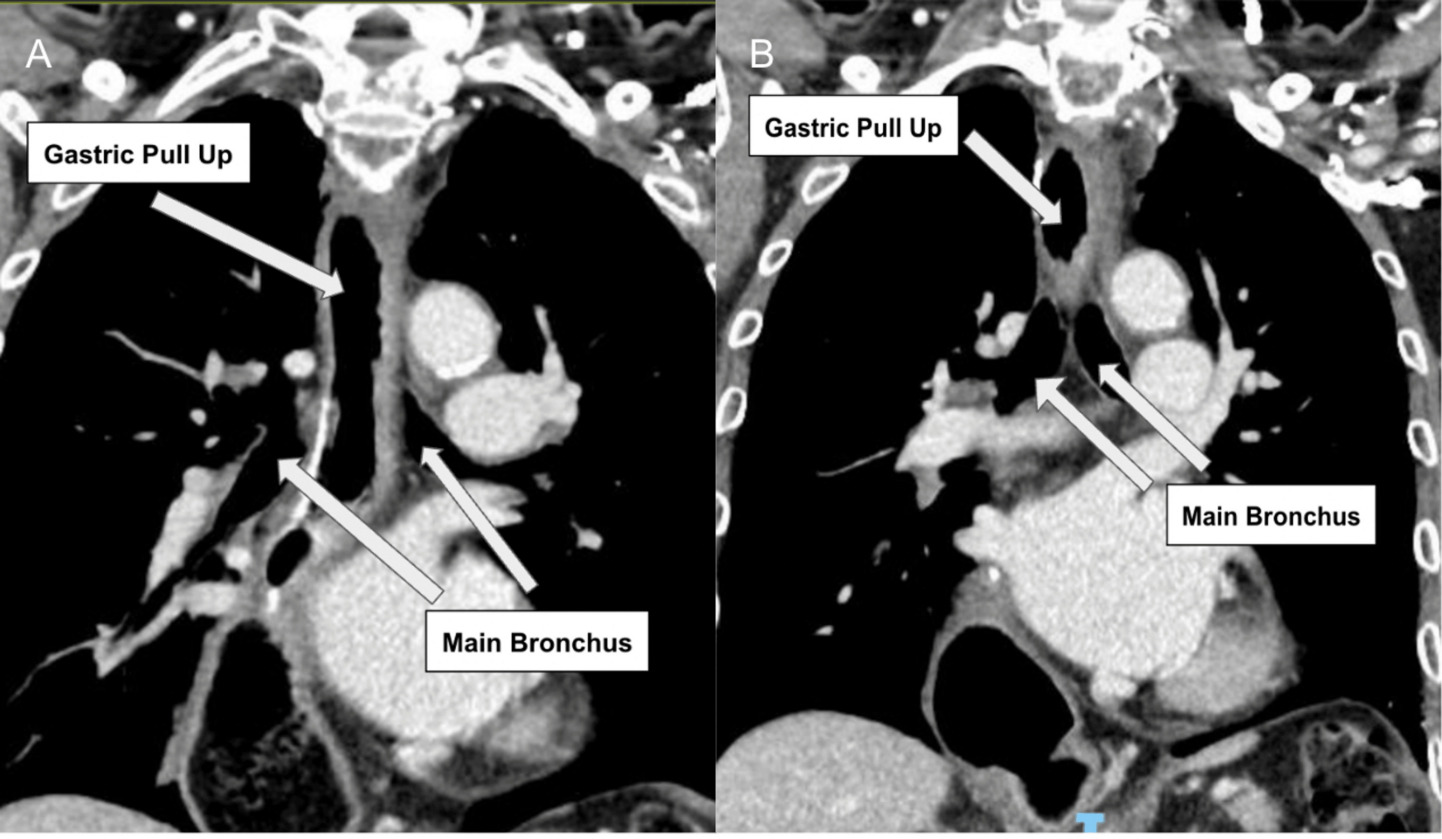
Coronal view of a computed tomography scan of the thorax in a patient with a previous total pharyngo-laryngo-esophagectomy, showing the gastric pull-up and the two main bronchi.
